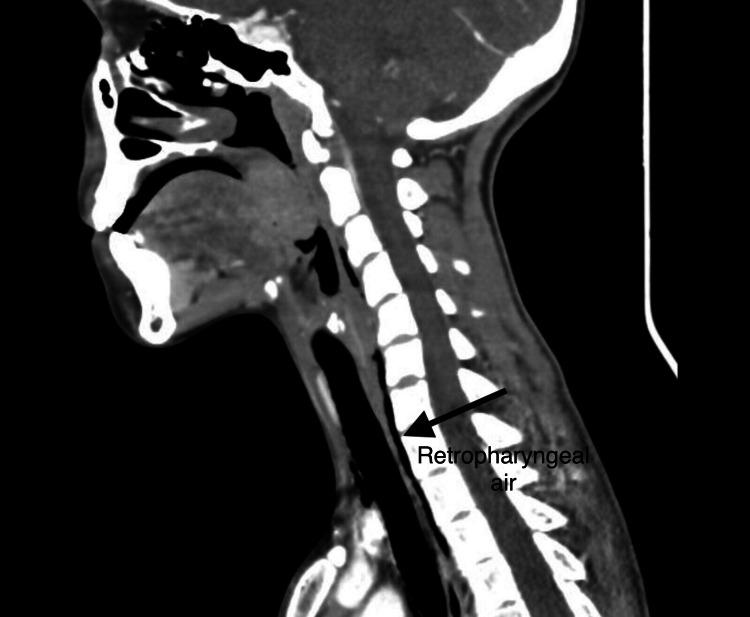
CT scan of neck and chest shows retropharyngeal air.
